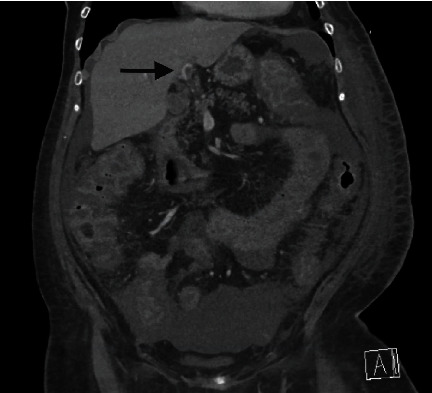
CT abdomen coronal view: arrow demonstrating acute left portal vein thrombosis.
